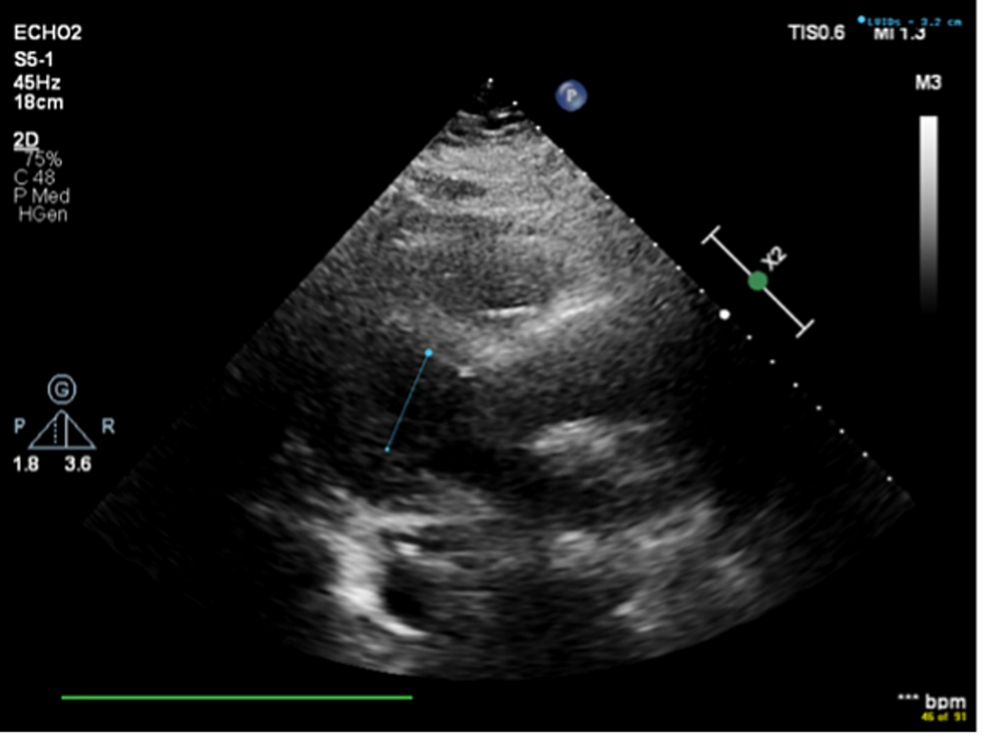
Limited 2D Echocardiogram showing Left Ventricular Internal End-Systolic Diameter (LVIDs) of 3.2 cm. (Parasternal long axis)
